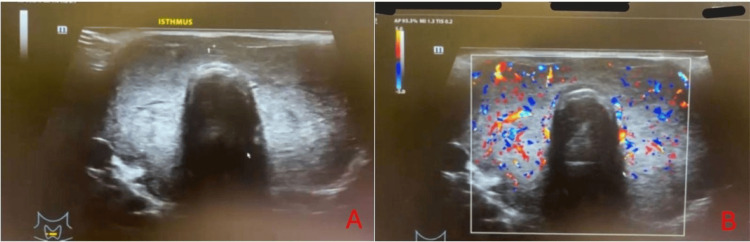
Ultrasound of the thyroid gland showing features of diffuse goiter (A) Transverse grayscale image demonstrating diffuse enlargement of the thyroid gland with heterogeneous echotexture involving the thyroid isthmus. (B) Color Doppler image demonstrating increased diffuse vascularity within the thyroid parenchyma, consistent with Graves’ disease
